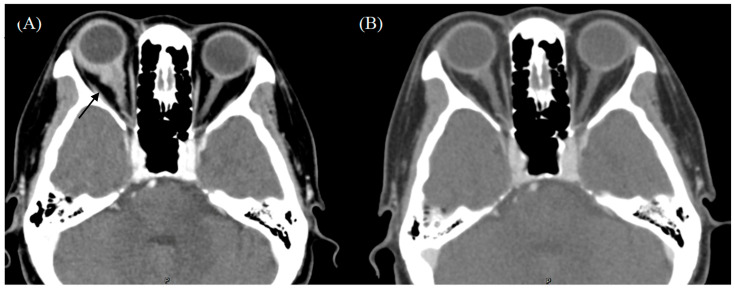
Computed tomography of the orbit demonstrated enhancing soft tissue density in the posterior margin of the right globe and along the right optic nerve. The right globe and optic nerve are compressed, causing exophthalmos (black arrow). (A) Before treatment. (B) After treatment.
